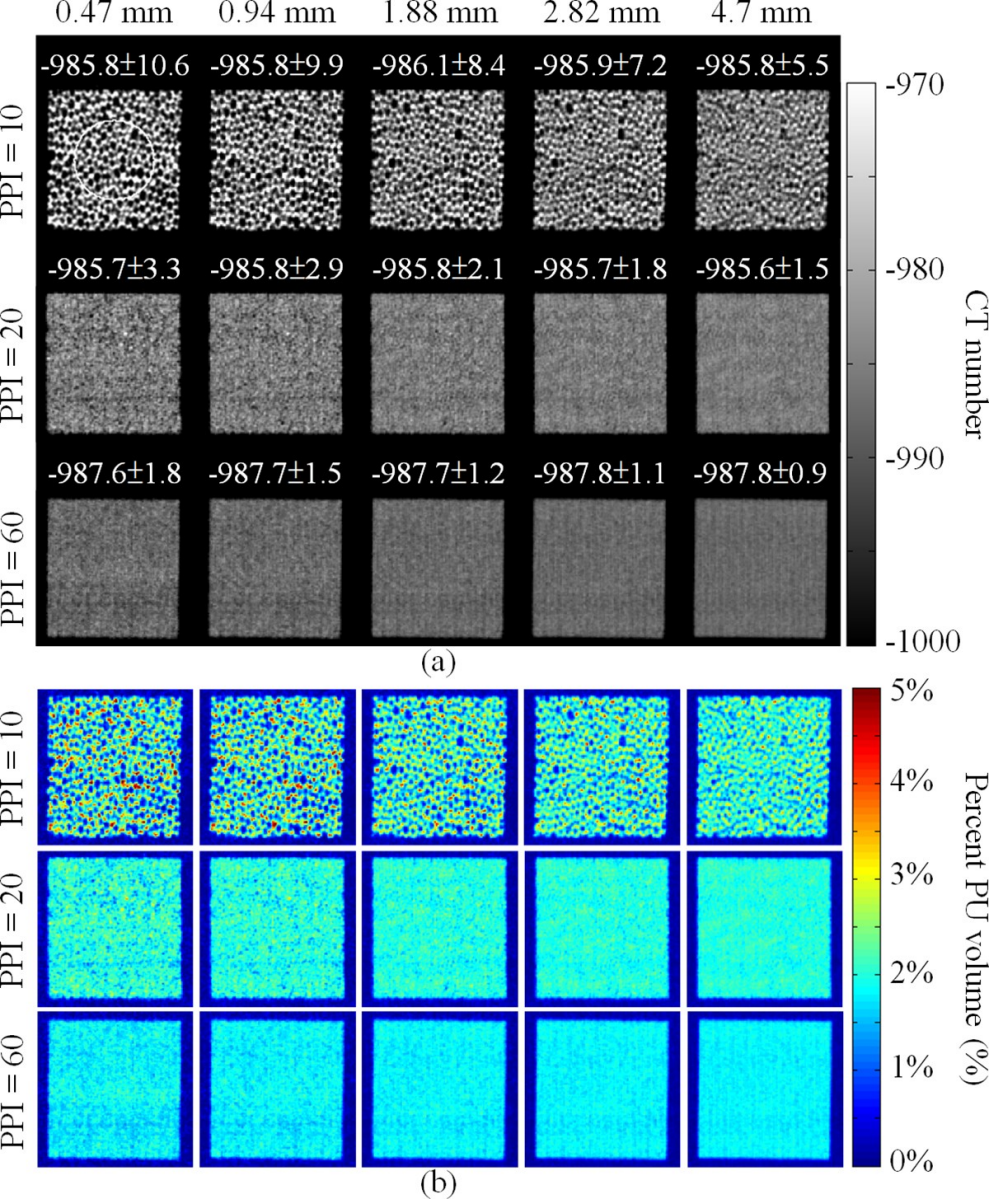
(a) CT images of the foams with slice thicknesses of 0.47, 0.94, 1.88, 2.82, and 4.7 mm with addressed mean CT numbers of the foams. (b) PU volume fraction maps calculated from (a) using the TCM with an air–PU submaterial pair. The white circle in (a) indicates the location of the ROI for the calculation of the mean CT numbers.
